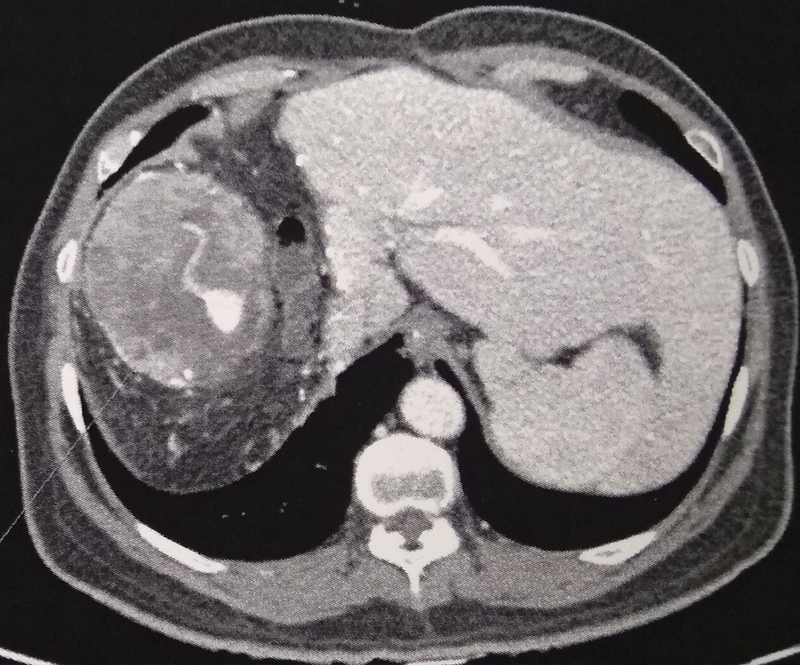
Contrast enhanced computed tomography axial image shows an intra-abdominal 10 × 9 × 9-cm lesion adherent to diaphragm and the operative bed along the cut surface of liver.
